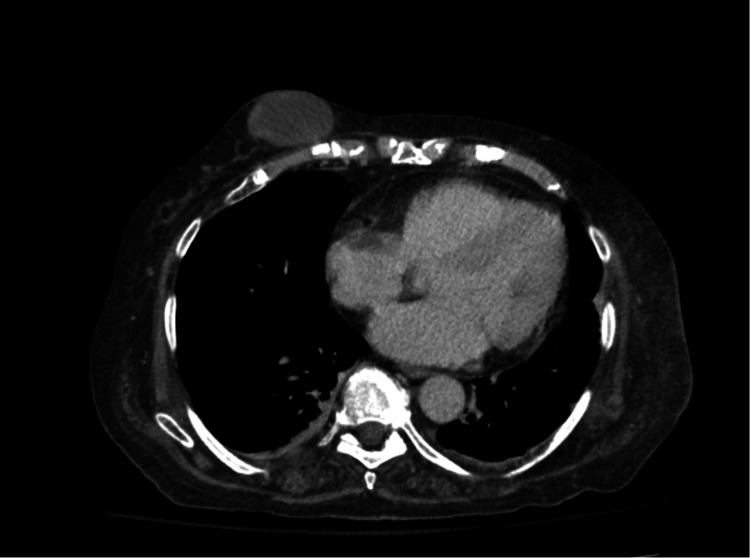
Chest CT of the patient at admission
